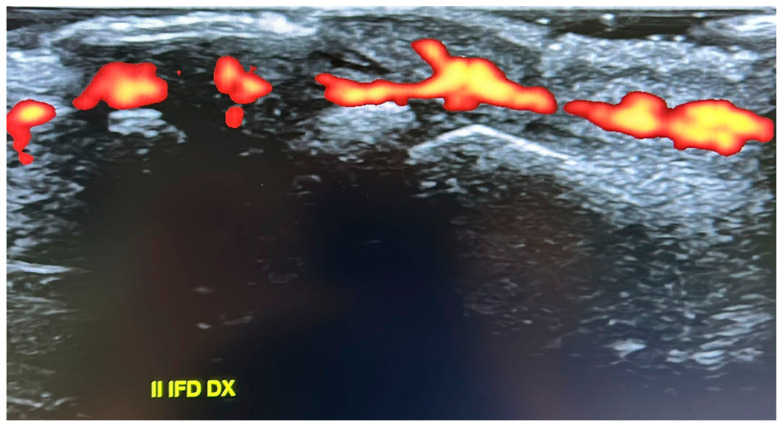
Ultrasound evaluation of the interphalangeal joints demonstrating a marked inflammatory signal on power Doppler imaging, consistent with active synovial inflammation.
